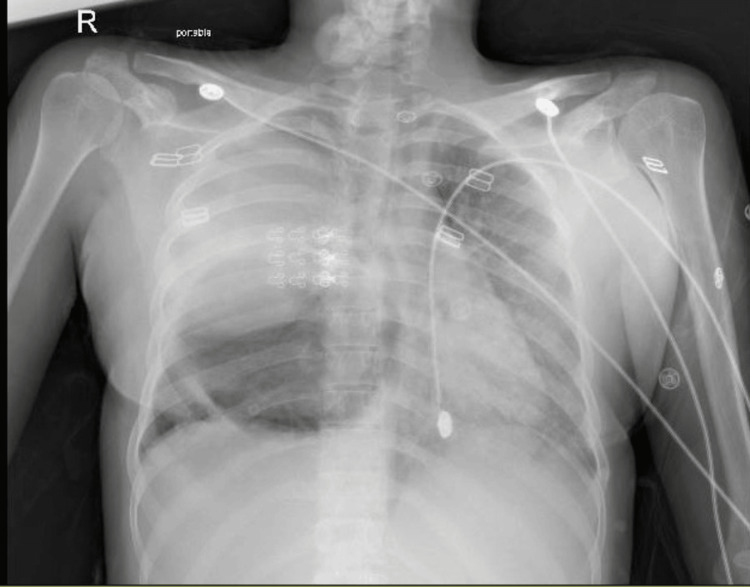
Chest X-ray, anteroposterior (AP) view: large cyst on the field of the right lung.
